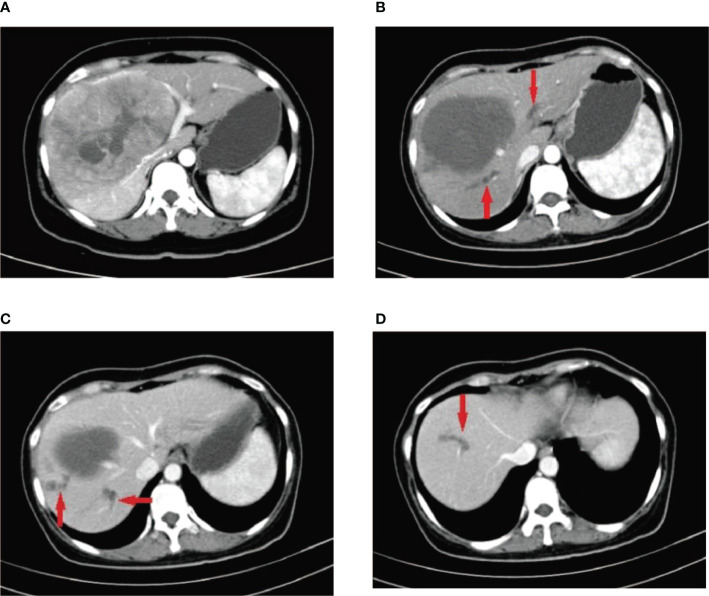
A 41-year-old female patient who was diagnosed with hepatitis B more than 10 years ago underwent CT examination due to epigastric pain, and CT findings indicated a large hepatocellular carcinoma in the right lobe of the liver (A). The patient underwent two DEB-TACE treatment sessions. After 6 weeks, CT reexamination revealed a significant reduction in necrosis of the liver tumor; however, multiple intrahepatic bile duct necroses were observed around the tumor without significant enhancement (B–D) (red arrow).
